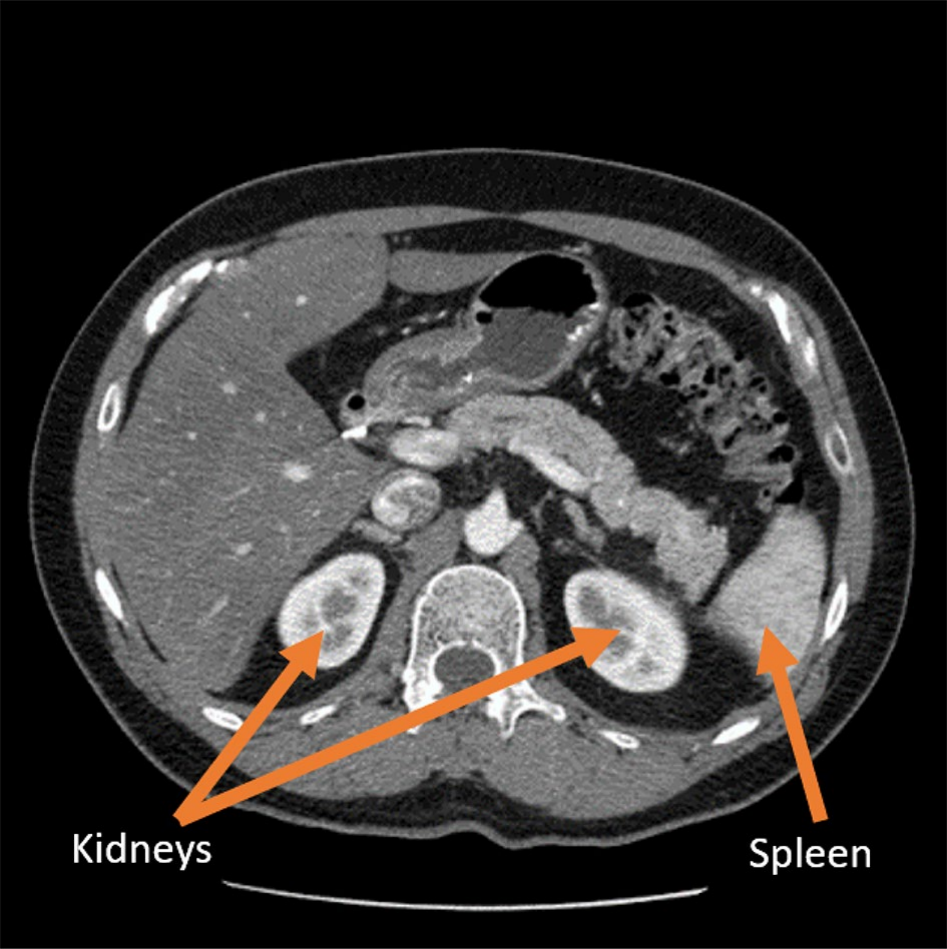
Example of a selected original CT section. The orange arrows indicate the labeled organs marked by an expert: Kidneys and Spleen, which are in the area of interest of the study.
